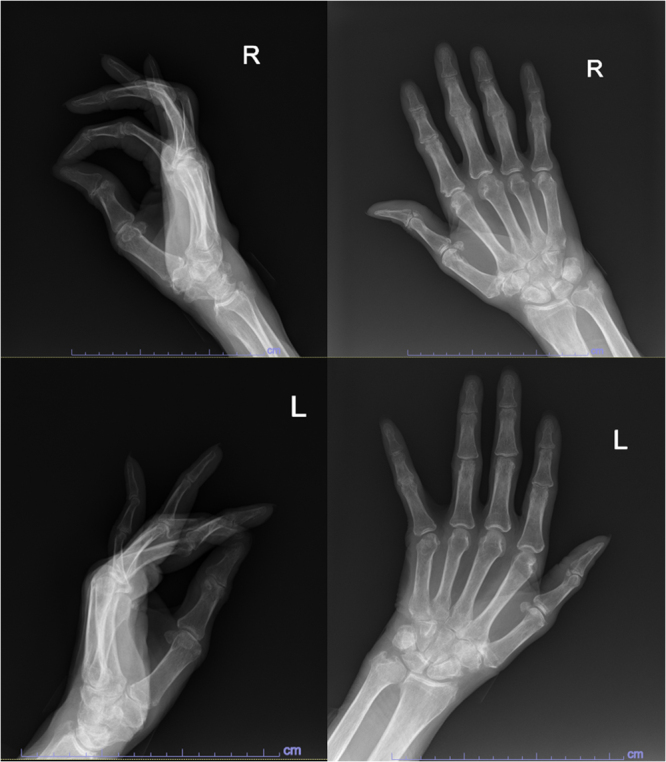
Hand X-ray showing erosive arthropathy involving the ulnar styloid, multiple metacarpophalangeal (MCP), and proximal interphalangeal (PIP) joints.
